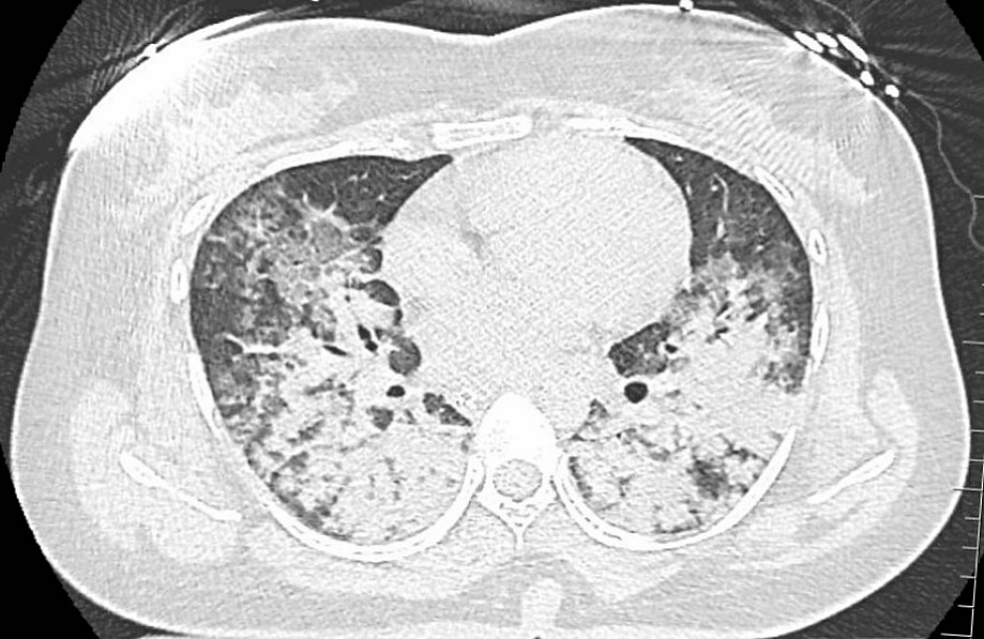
CT chest. CT chest showing bilateral pulmonary consolidation and ground glassing. CT: computed tomography.
